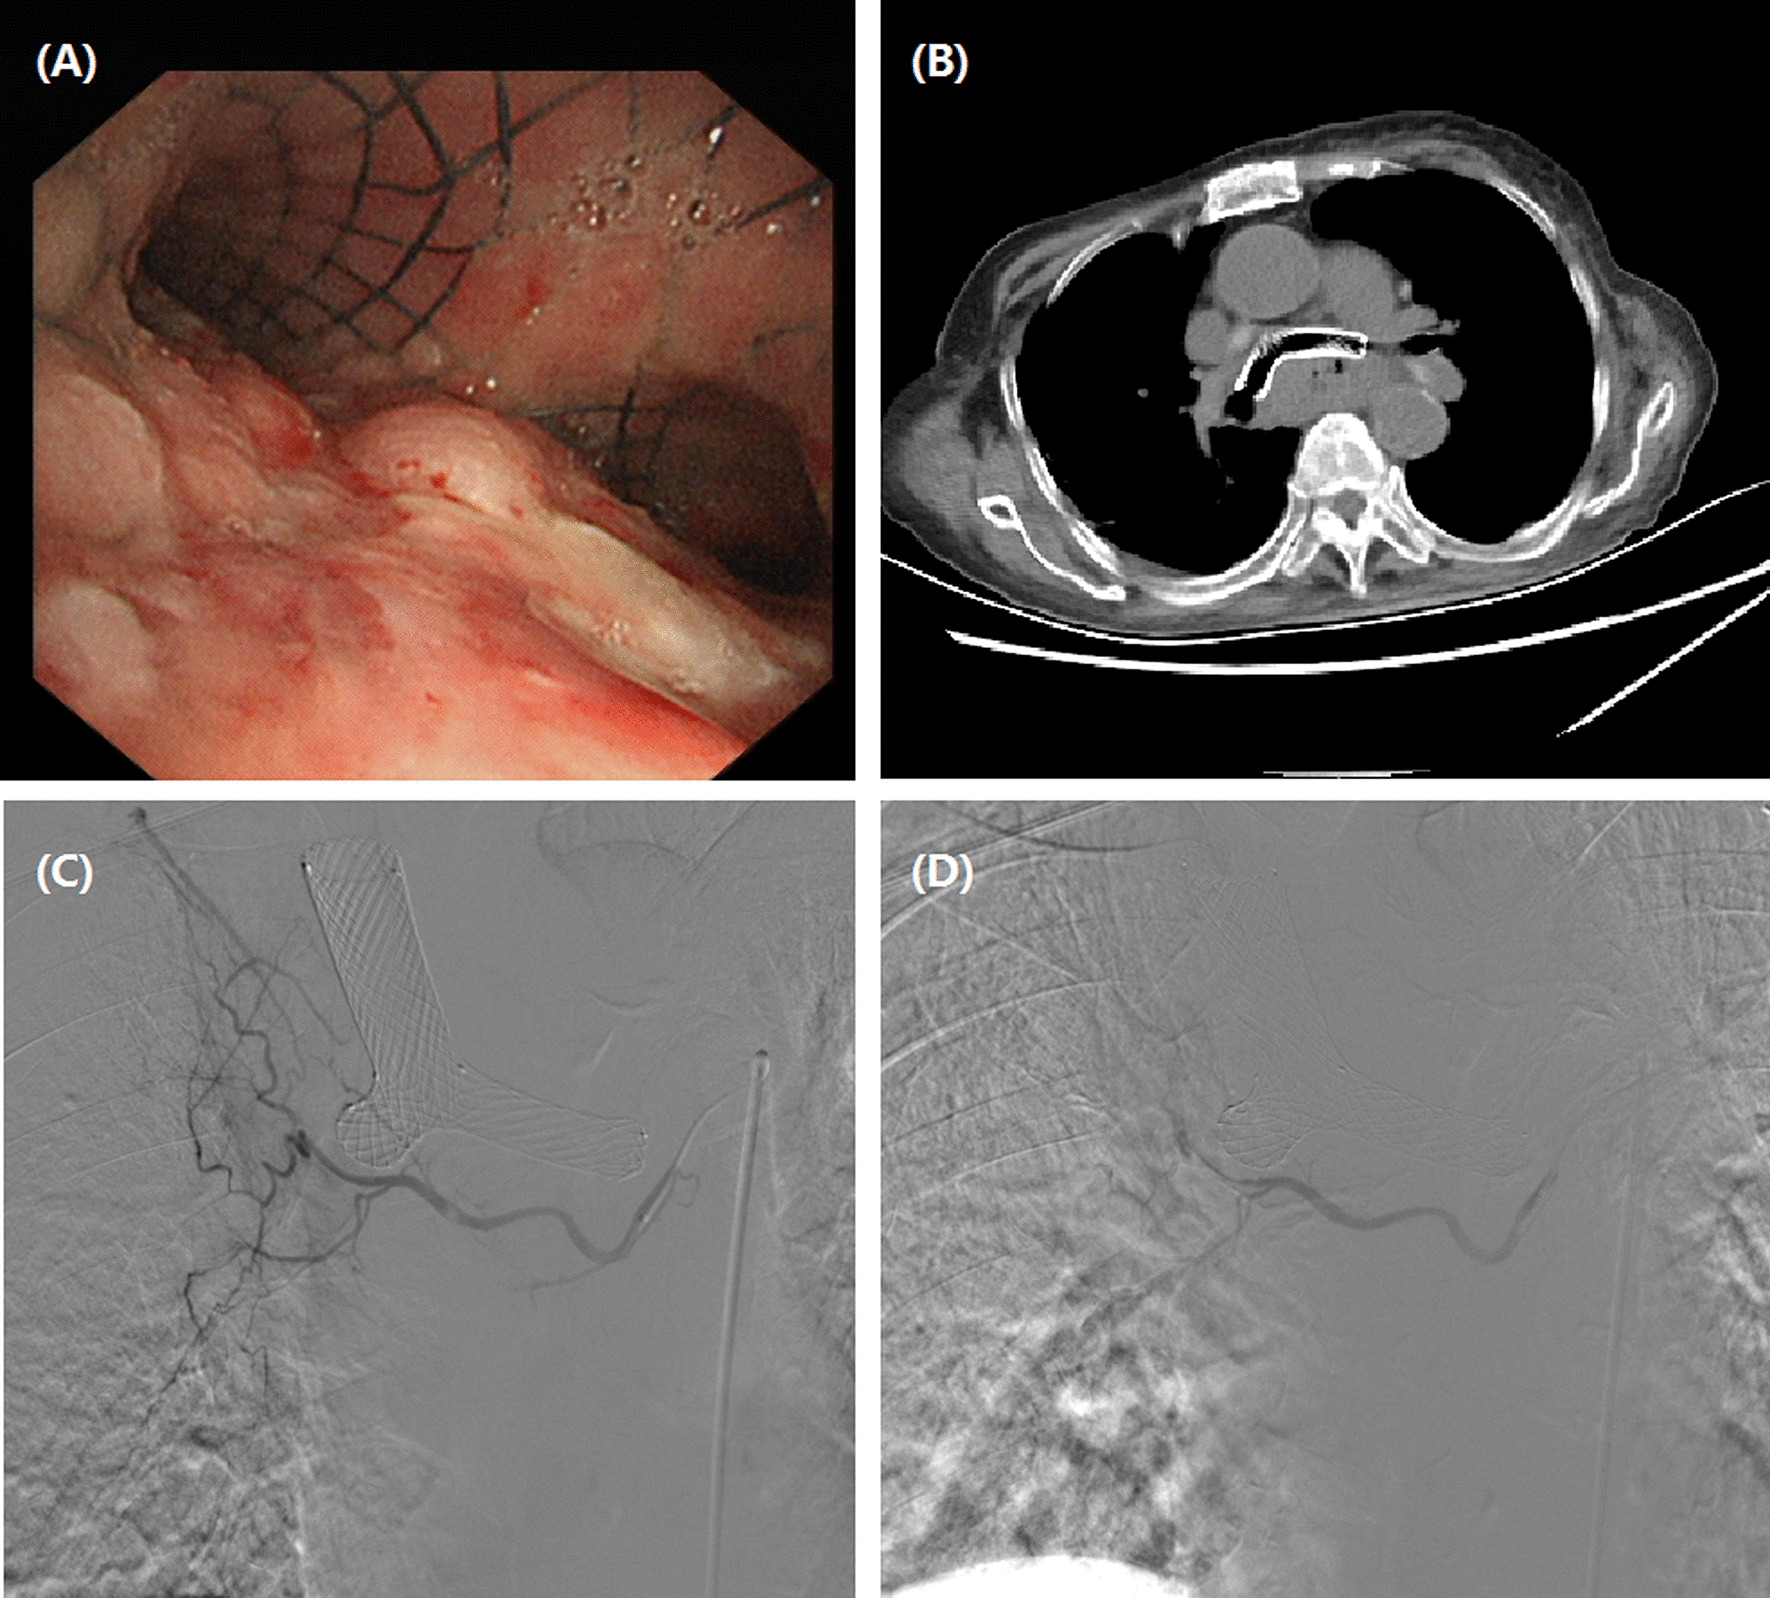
Images of a 75-year-old woman with esophageal cancer in upper and middle esophagus. aBrancheoscopy shows esophageal tumor ingrowths into tracheal wall surrounding the airway stent. bCT scan shows an obvious thickening of esophageal wall and invasion into tracheal wall surrounding the airway stent. dThe right bronchial artery is super-selectively catheterized by a microcatheter. eTumor-feeding artery was embolized using doxorubicin loading-CalliSpheres beads
